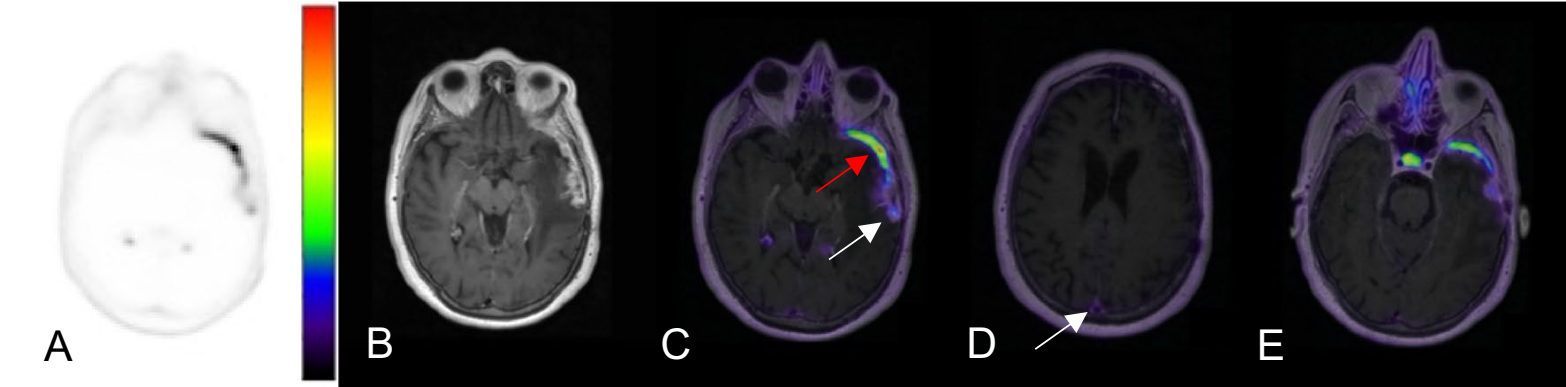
Axial images of [^68^Ga]-DOTATATE PET AC (A), 3D T1 post-gadolinium MR (B), fused PET/MR T1 (C–E) windowed SUV 0–15. This representative patient from the cohort is a 70-year-old female with a history of WHO grade II meningioma s/p resection and proton therapy 6 years prior to imaging and an additional proton therapy a year prior. The fused PET/MR images demonstrate a lesion suspicious for a meningioma in the left anterior temporal pole with SUV of 13.6 (C, red arrow). The more posteriorly located enhancing lesion (C, white arrow) demonstrates SUV of 4.5 and was suspicious for post-treatment change, given that the SUV of the superior sagittal sinus was 2.3. The subsequent resection and biopsy of the two lesions a year later confirmed the suspected diagnosis of recurrent meningioma and radiation necrosis, respectively. The superior sagittal sinus (D, arrow) demonstrates SUV of 2.3 and the pituitary gland (E) demonstrates SUV of 12.4.
